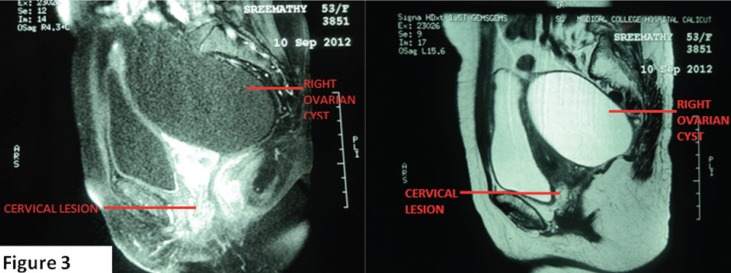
MRI pelvis showing well-defined iso-hyperintense lesion in the cervix (2.8 x 2.4 cm) and a large simple right ovarian cyst hypointense on T1Wand hyperintense on T2W.
